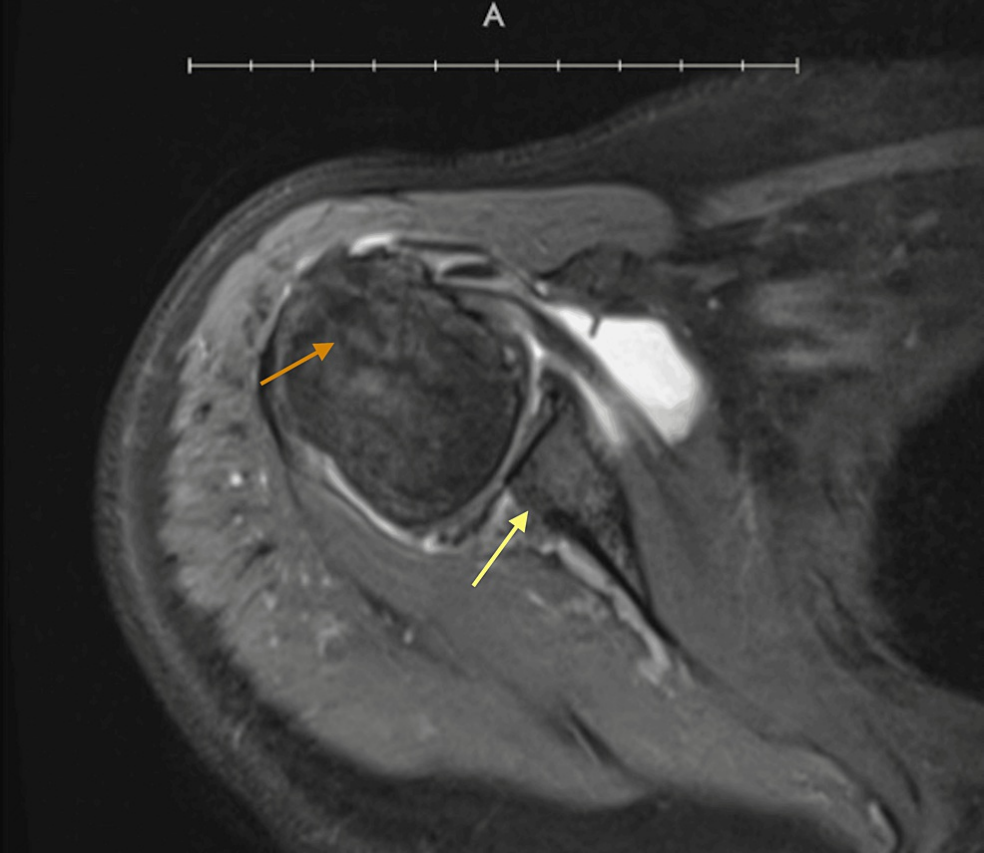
Axial MRI without contrast Chondral thinning of the humeral head (orange arrow). Full-thickness cartilage loss at the posterior inferior glenoid and subchondral cystic change is also noted (yellow arrow).
